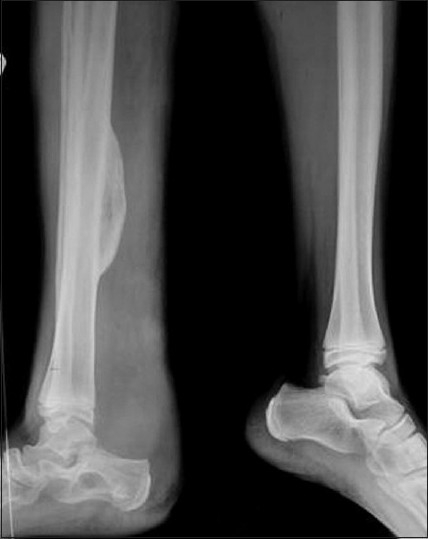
Conventional radiograph of the bilateral lower limbs shows disparity in limb length. The right limb appears relatively longer and shows soft tissue hypertrophy, periosteal and endosteal thickening (involving middle 1/3rd shaft of the tibia), scalloping of planter surface of right calcaneum and erosion of articular surfaces of the right talocalcaneal joint
